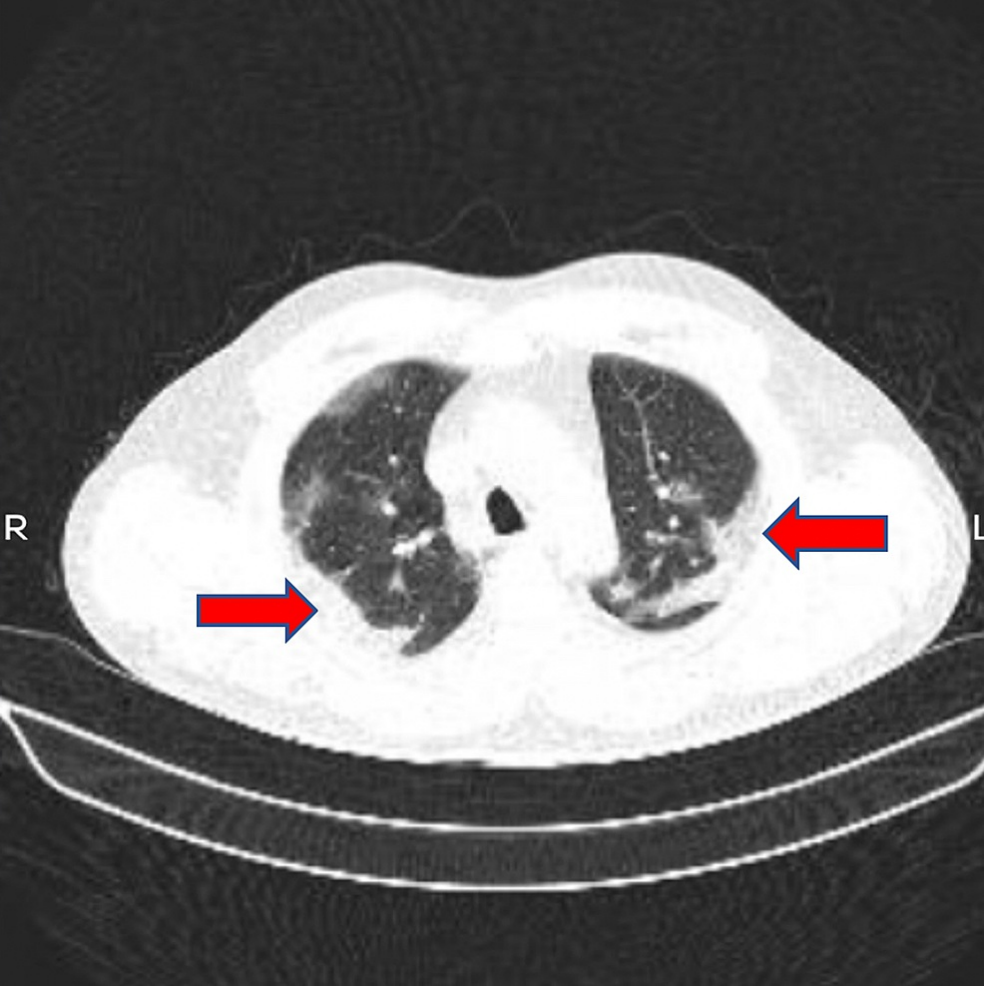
Showing HRCT chest with bilateral ground glass opacities predominantly in the lower lobes signifying COVID pneumonia. HRCT: High-resolution computerized tomography.
